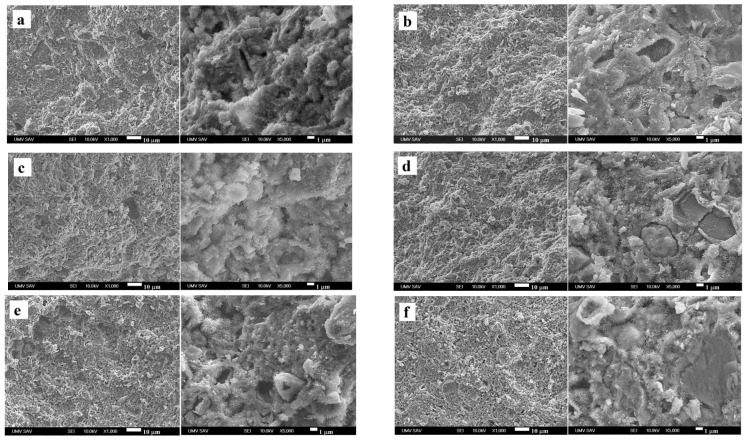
Microstructure of fractured cements after 7 days of hardening in SBF at 37 °C: CT (a), CT1Si (b), CT2Si (c), CT5MP (d), CT5MP1Si (e), CT5MP2Si (f).
